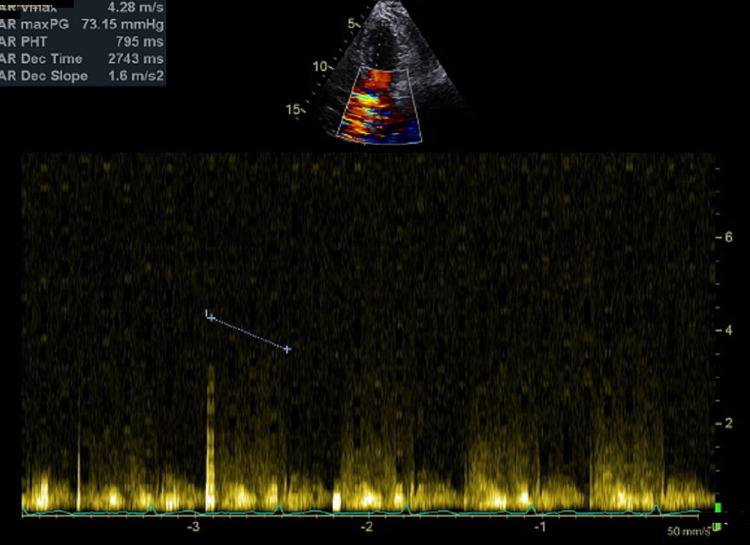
Continuous wave Doppler across the aortic valve measuring pressure half time showing mild aortic regurgitation.
